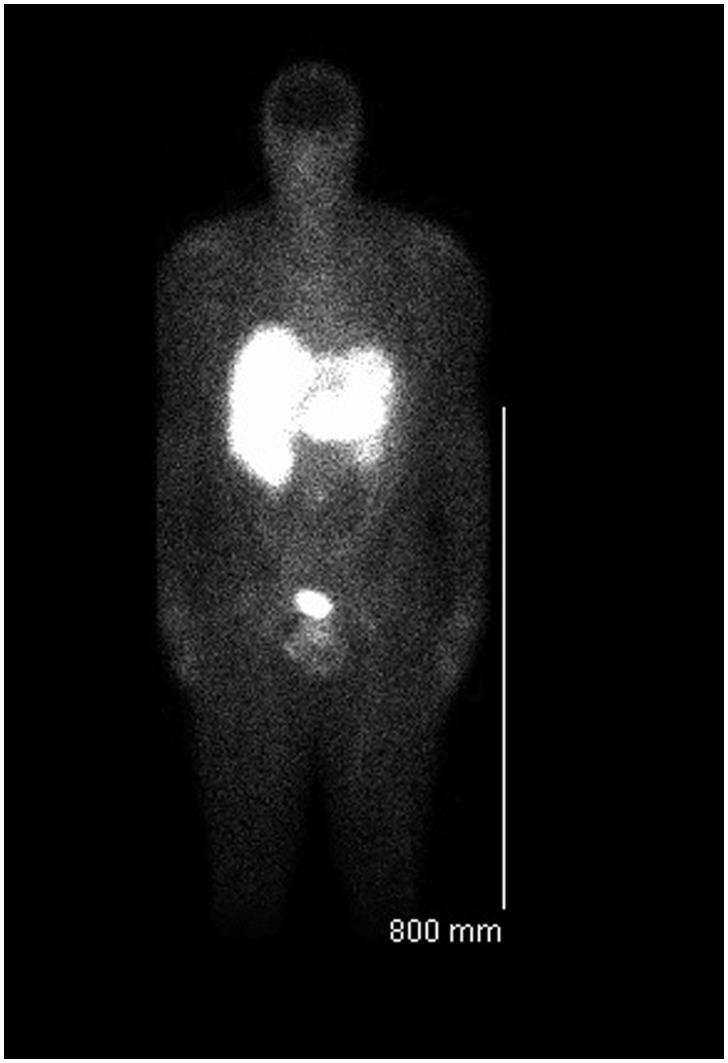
Somatostatin receptor scintigraphy image demonstrating radiotracer uptake in multiple liver metastases.
